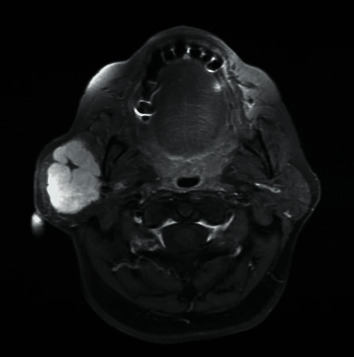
An axial MRI image demonstrating a lobulated lesion centered within the superficial lobe parotid gland (right side).
